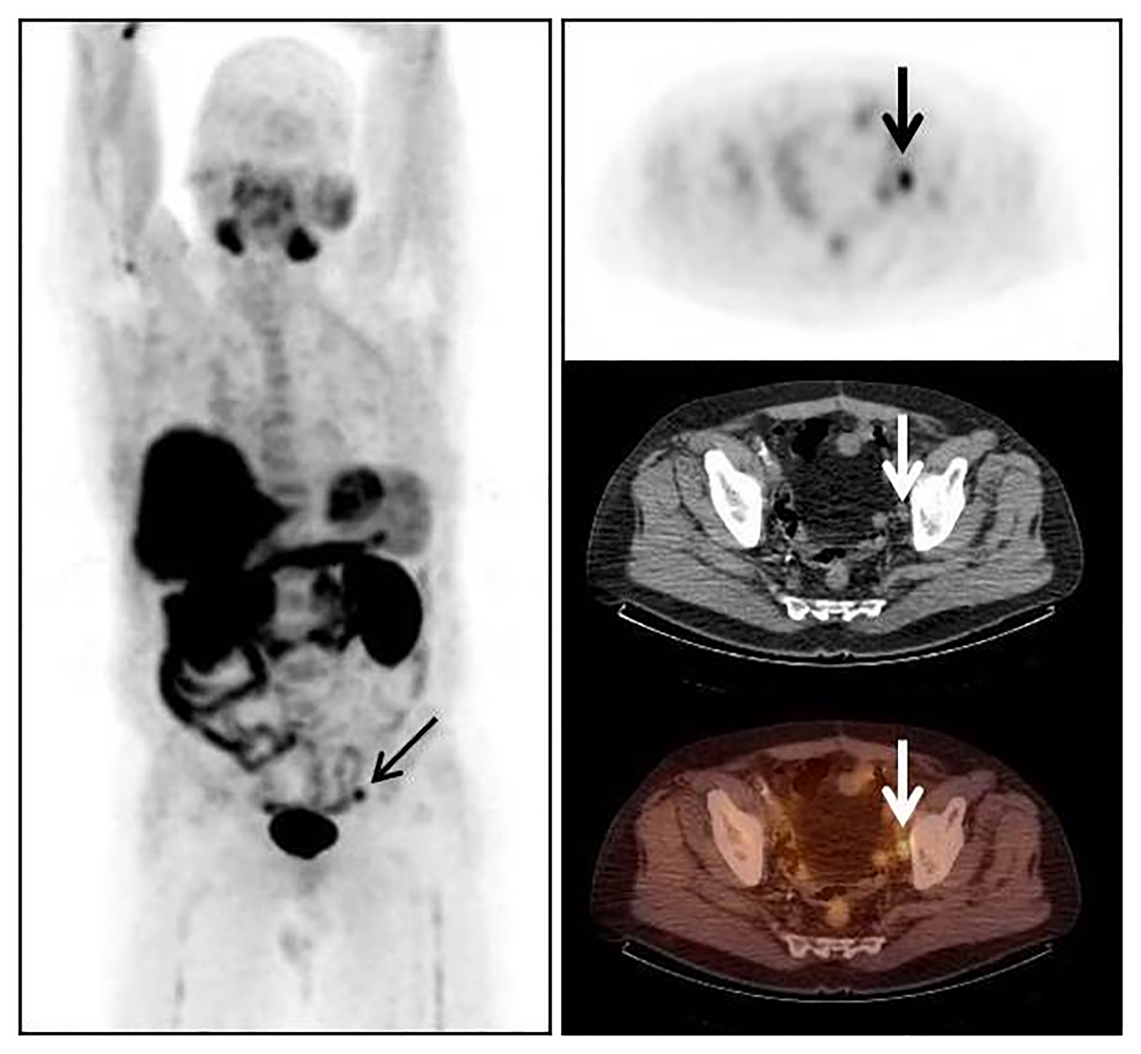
64y old patient with prostate cancer Gleason score 8 treated 6 years ago by radical prostatectomy. PSA serum level at the time of FCH PET/CT 3.5 ng/mL. Intense FCH uptake by left external iliac lymph node evocative of prostate cancer recurrence. Scheduled watchful waiting replaced by complete androgen blockade leading to complete remission of disease according to follow-up data.
